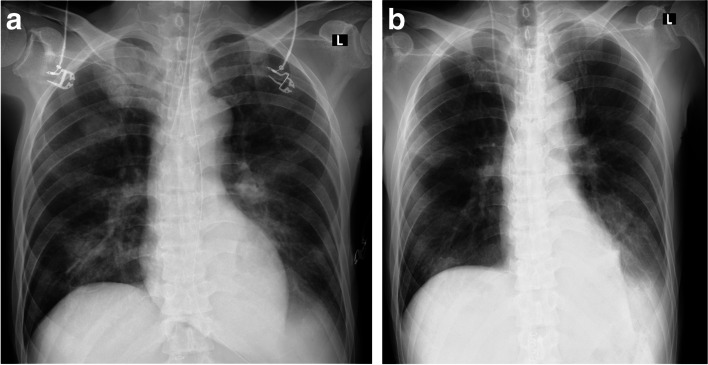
Opacities in the right lower lung lobe were found immediately after surgery. Chest radiograph on POD 10. Dullness at the left costophrenic angles remained, but the opacities in the right lower lung lobe were improving after treatment. POD, postoperative day
